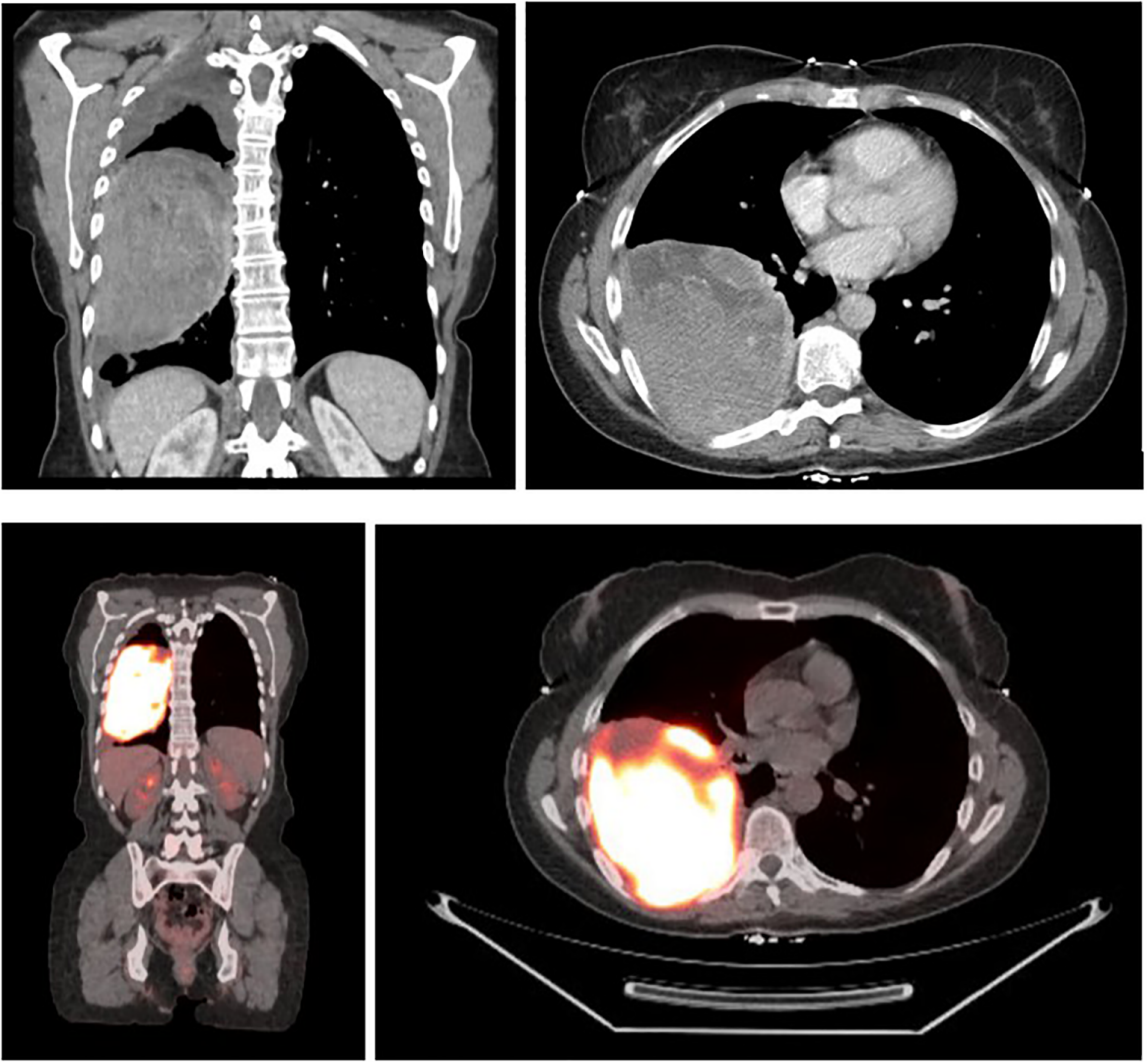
CT chest 5/25/2022 demonstrating a 12.0 x 9.1 cm right lower lobe mass, with FDG F-18 PET 6/3/2022 noting avidity and no evidence of metastatic disease, SUV max 24.6.
